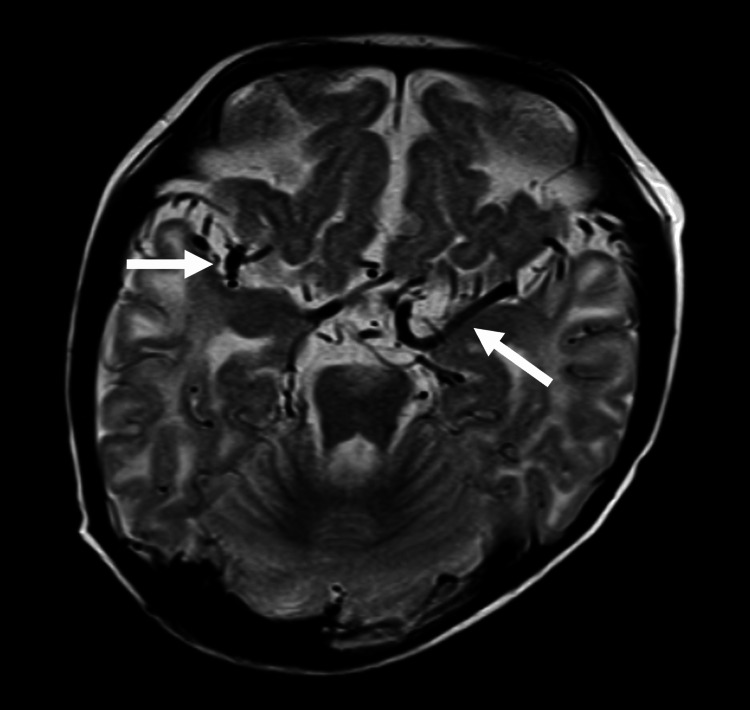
Case 1: T2-weighted image. Tortuosity and bending of the bilateral middle cerebral arteries are noted in the Sylvian fissure (arrows).
